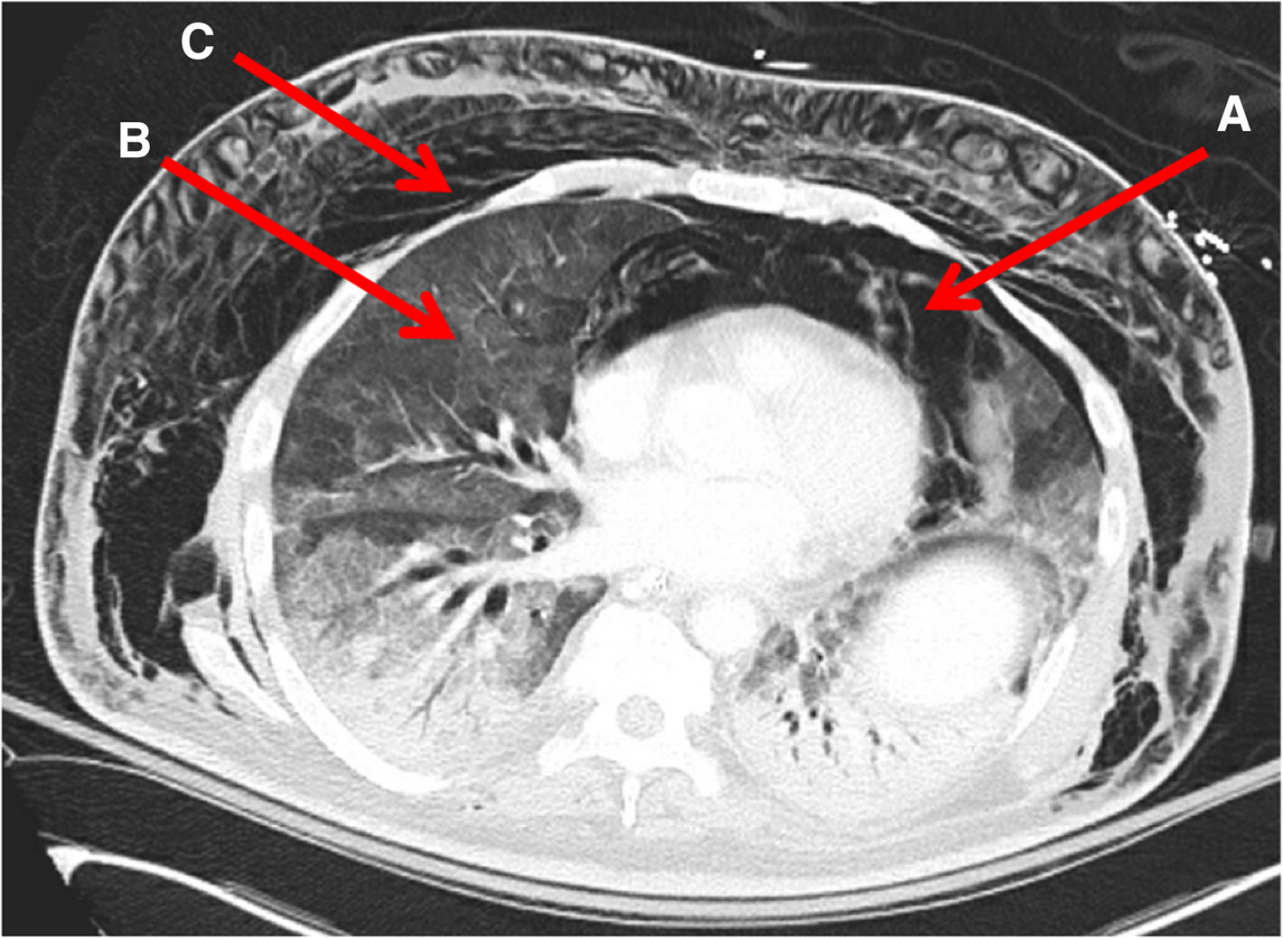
Computed Tomography of Chest, Sagittal View. a Severe pneumomediastinum extending from lower neck, surrounding great vessels off aortic arch and anterior portion of the heart. b Diffuse severe ground glass opacities in bilateral lung fields with superimposed dense consolidations with air bronchograms. c Extensive subcutaneous emphysema involving all fascial compartments
